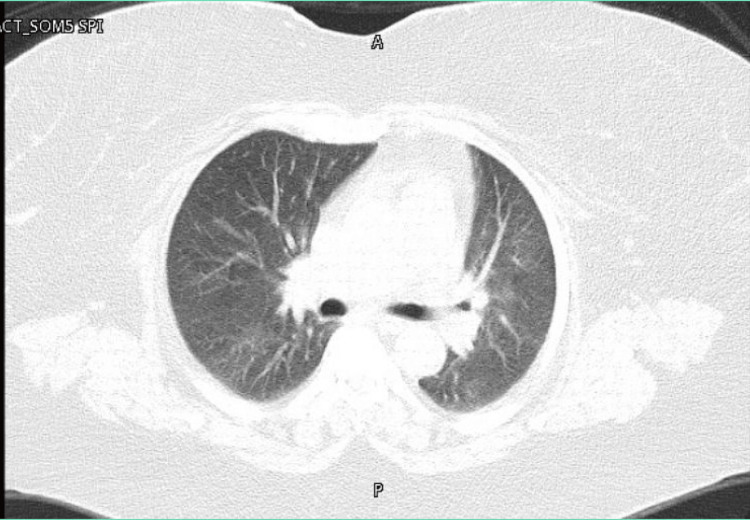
Follow-up chest CT at eight weeks showed complete resolution of the previously observed changes
